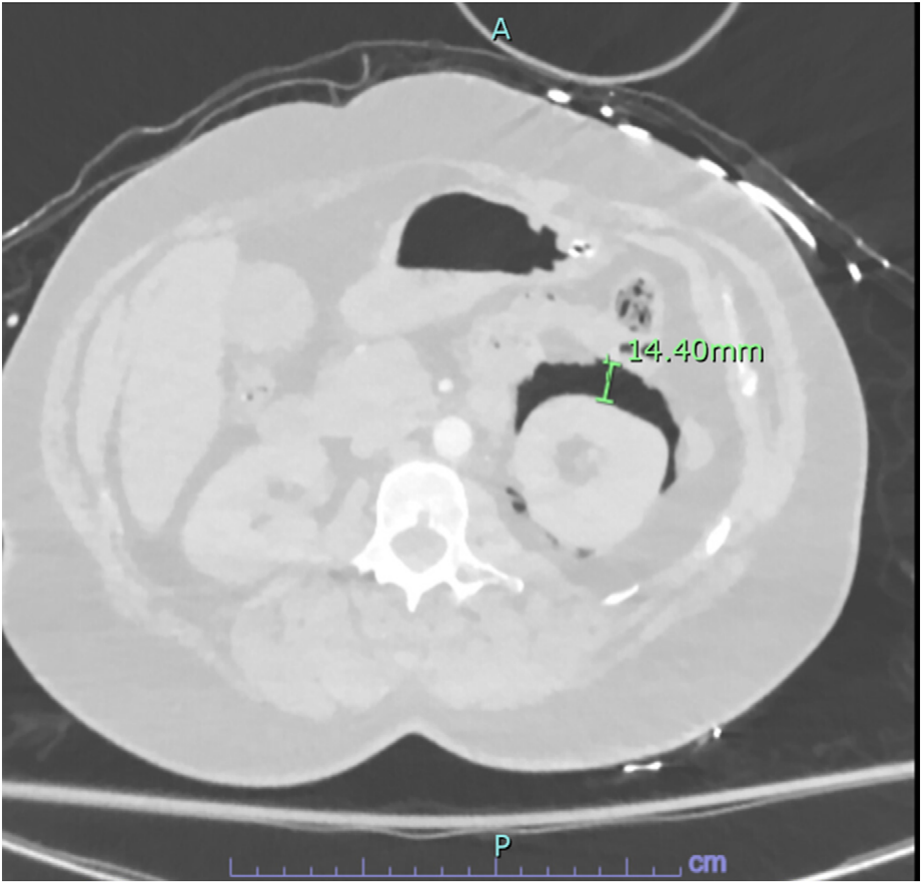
Image from patient’s computed tomography (CT) with pulmonary embolism protocol performed on the day of presentation revealing air in the left perinephric space, which is likely related to retroperitoneal extension of air from the patient’s pneumomediastinum as a CT of the abdomen and pelvis was performed and revealed no other acute traumatic injuries in the abdomen or pelvis that could account for the free air.
